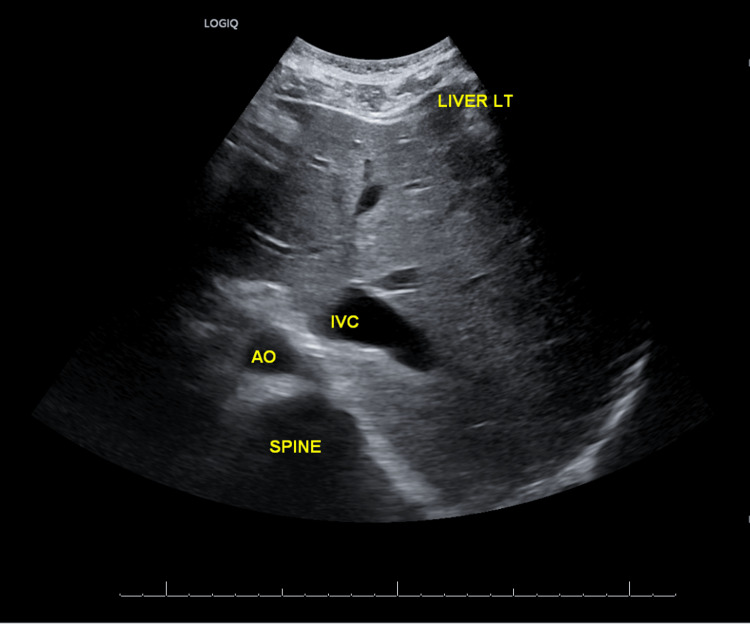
Abdominal Ultrasound Demonstrating Mirror-Image Organ Orientation in Situs Inversus Totalis Subcostal ultrasound view demonstrating the liver (LIVER) and inferior vena cava (IVC) positioned on the left of midline and the aorta (A) on the right, confirming mirror-image abdominal organ orientation consistent with situs inversus totalis (SIT). The spleen was also visualized in the right upper quadrant during scanning but is not included in this image.
